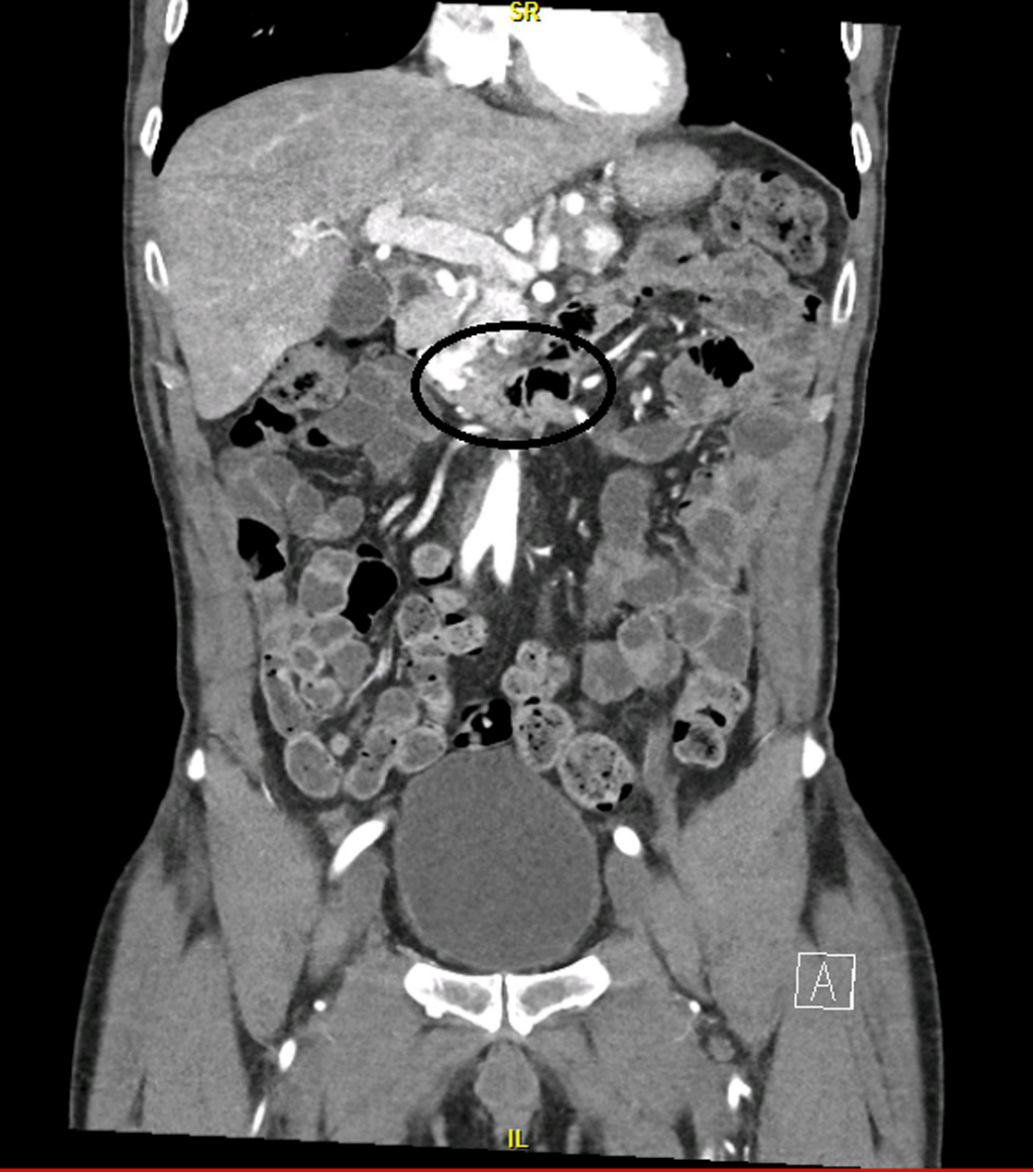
CT of the abdomen showing new intraluminal metastasis invading into the duodenum arising from the largest pancreatic head metastasis measuring 2.5 cm.
